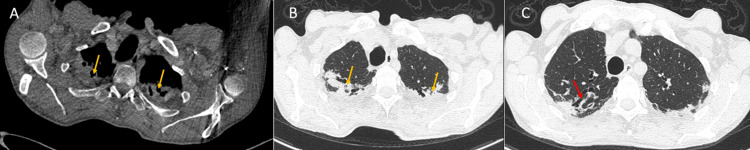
Coronal view of thoracic computed tomography with bone window study (A) and parenchymal window study (B and C) showing apical pleural thickening and subpleural consolidations (yellow arrows), and traction bronchiectasis (red arrow).
